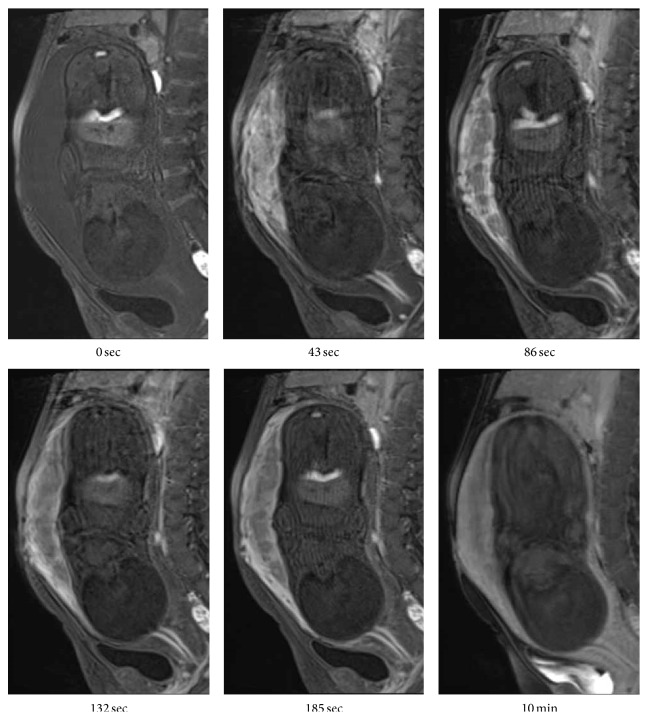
Dynamic contrast-enhanced-MRI using gadolinium at 37 weeks of gestation revealed that the myometrial lesion was enhanced slowly and showed homogeneous enhancement even on a 10 min delayed image, whereas the placenta demonstrated marked arterial enhancement and subsequent rapid washout.
